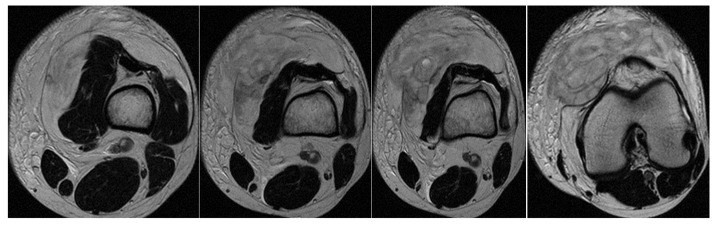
Knee MRI: axial T2 acquisition.
